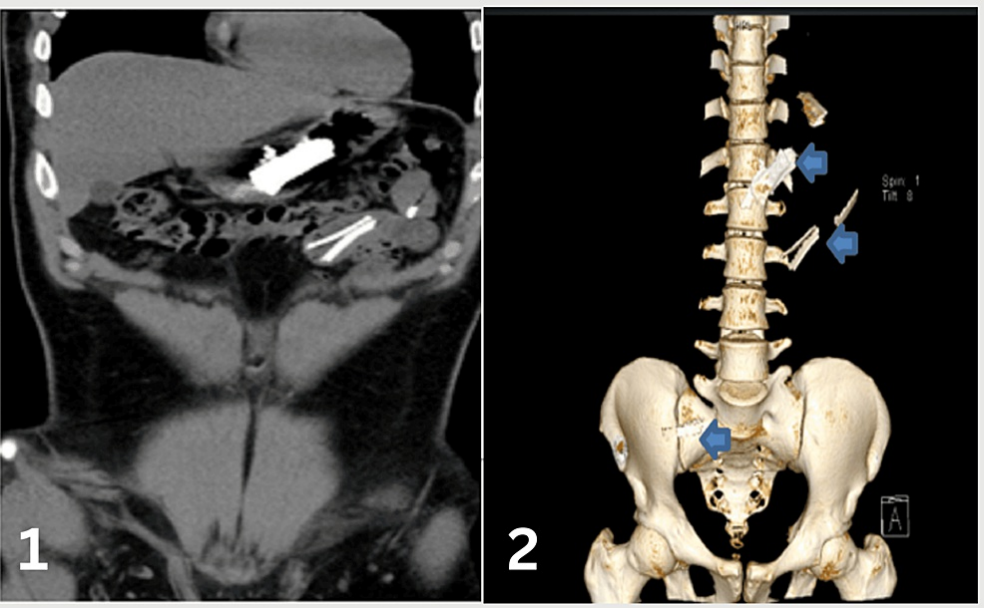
Abdomen computed tomography (CT) showed sharp objects in the stomach and small bowel (1) and a 3D bone window (2)
